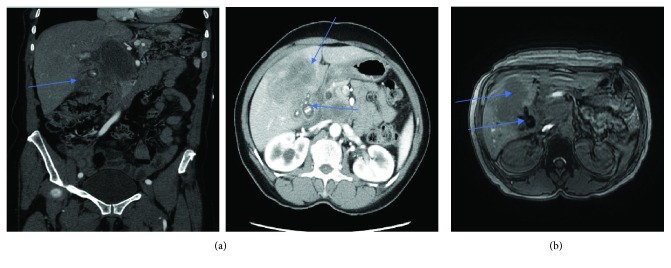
(a) CT of the abdomen coronal and axial views. A complex mass with foci of calcification in gallbladder fossa and 9 cm heterogeneous mass in the right hepatic lobe. (b) MRI of the abdomen axial view: large gallbladder complex mass of 5 × 4 cm with engulfed calcifications and stones extending into the liver. 9 cm liver mass with some central necrosis and calcifications and loss of clear fat plane separation between the gallbladder and right liver, associated with large hilar lymphadenopathy (4 × 5 cm) and compressive effects on the distal biliary system.
